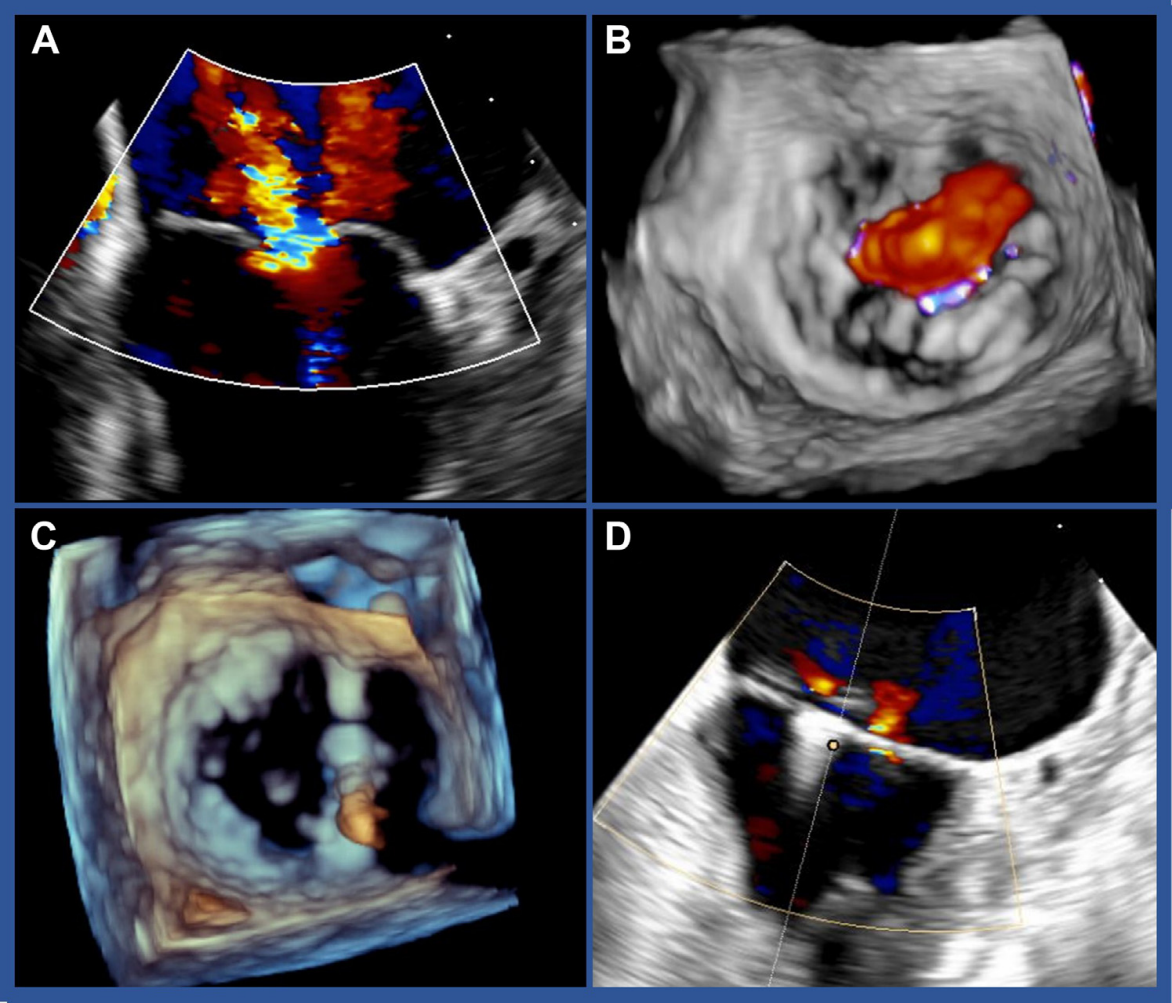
Evaluation and Treatment of Mitral Regurgitation Using a PASCAL Ace Device (A and B) Severe mitral regurgitation according to echocardiography in 2 and 3 dimensions. (C) Implantation of a PASCAL Ace slightly medial. (D) Final result.
